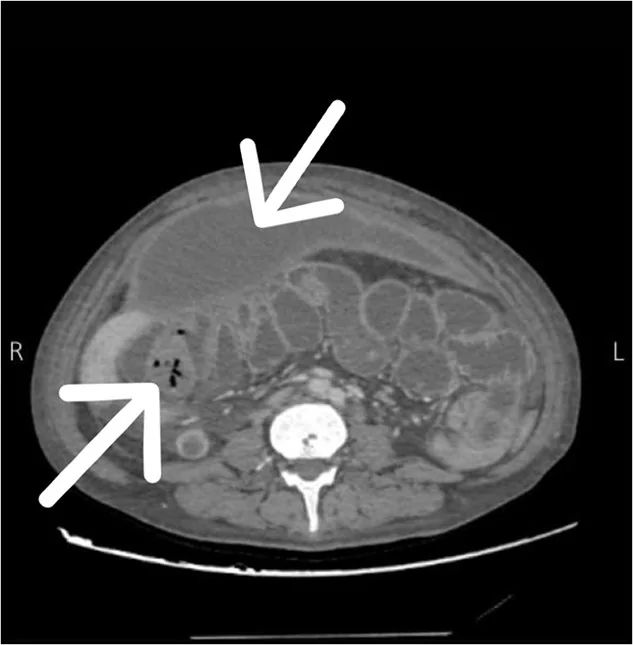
Contrast-enhanced CT scan of the abdomen; the upper arrow highlights diffuse peritoneal thickening characteristic of EPS, while the lower arrow indicates the specific site of the acute “closed-loop” intestinal obstruction.
